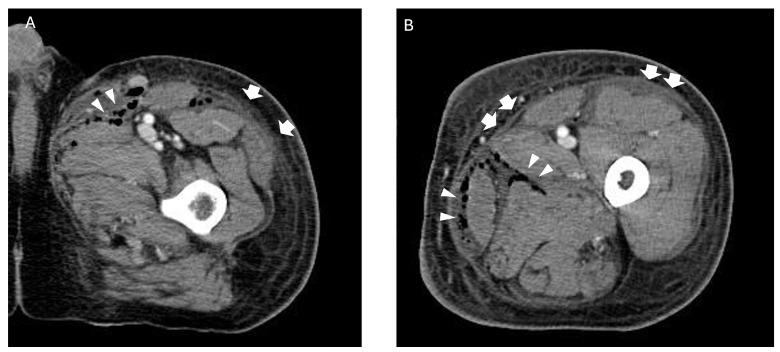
Axial CT images (A,B) of lower extremity show soft tissue edema, thickening fascial (arrowheads) and internal gas (arrows) tracking along superficial and deep fascial planes, consistent with necrotizing fasciitis.
